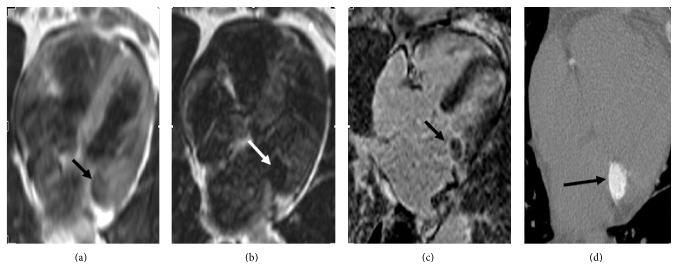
T1-weighted (a) and T2-weighted (b) turbo spin echo images show hypointense round mass-like lesion (black and white arrows). Delayed postcontrast cardiac MRI image (c) shows peripheral enhancement (black arrow). Multislice noncontrast computed tomography image (d) shows round calcification (black arrow) located in mitral annulus.
